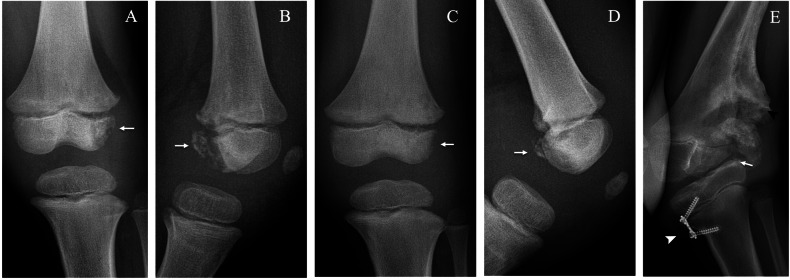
Left knee avascular necrosis in the brother of the patient shown in Fig. 8 at 5 years of age. Anteroposterior (AP) (A) and lateral (B) radiographs at injury show fragmentation of the lateral femoral condyle (arrow). AP (C) and lateral (D) radiographs at 21 days show partial healing of the lesion (arrow). AP radiograph at 9 years of age (E) shows progression of the bone resorption in the lateral condyle (arrow) with abundant callus formation (black arrowhead) after medial tibial hemi-epiphysiodesis (white arrowhead)
